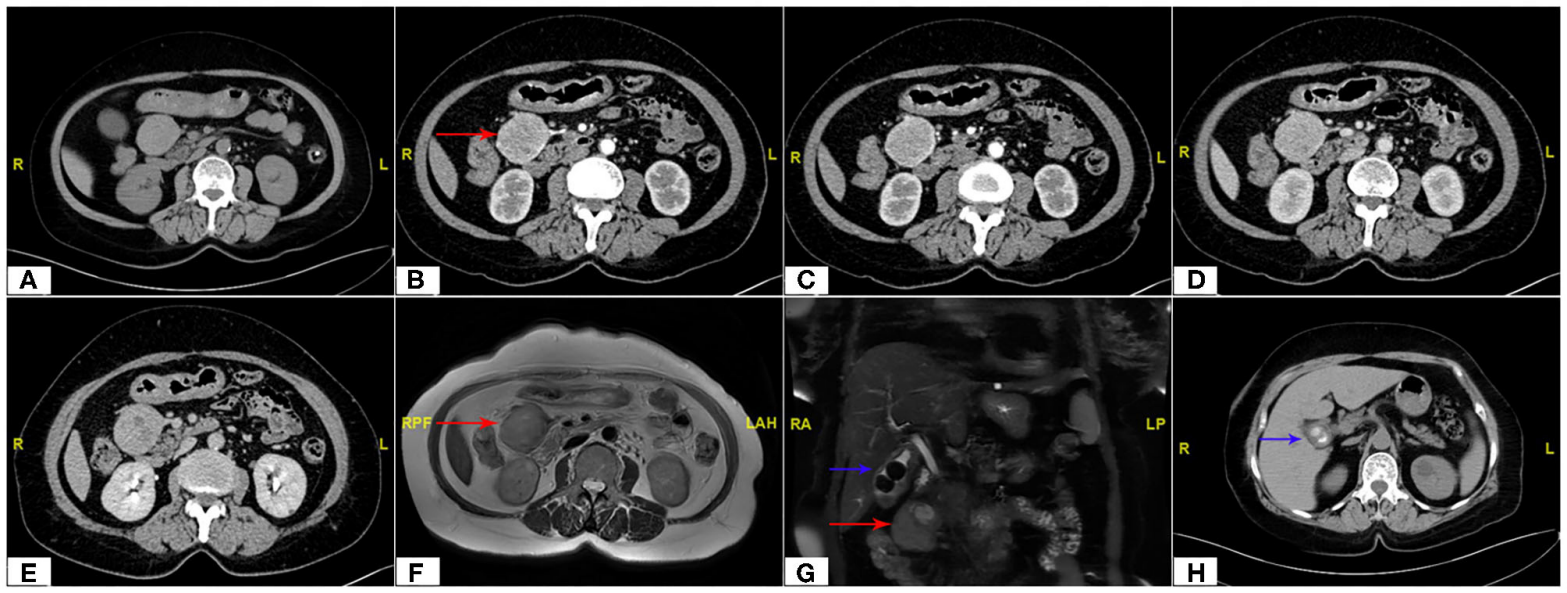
Abdomen CT scan showing the maximum cross section and the main blood supply vessels of the tumor (red arrow). (A) Non Contrast, (B,C) Arterial Phase, (D) Venous Phase, (E) Delay Phase; MRI imaging (F,G) of tumor (red arrow) and gallstones (blue arrow); CT scan of gallstones (H).
